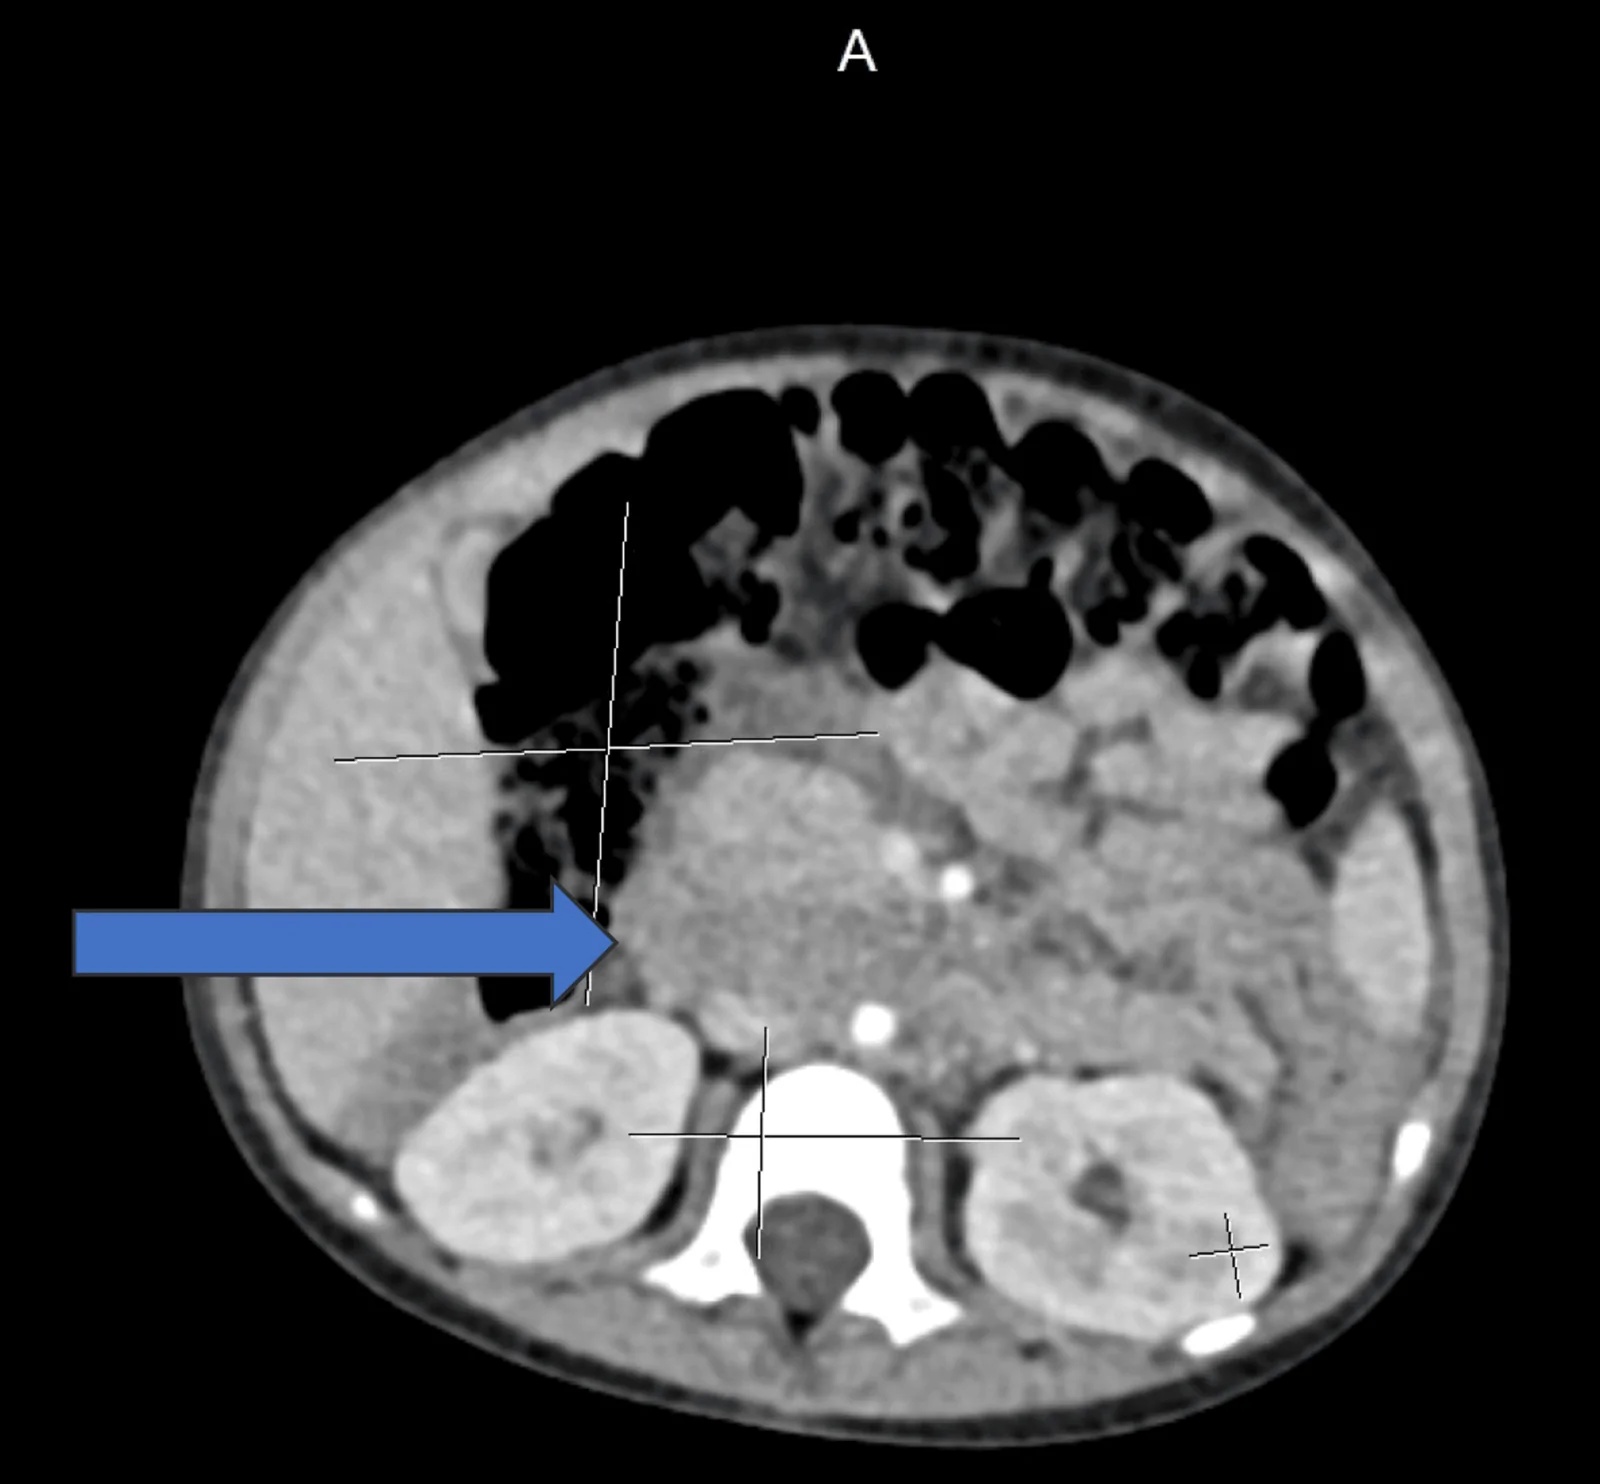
Contrast-enhanced CT scan demonstrating the primary intraabdominal mass during inpatient hospitalization CT: computed tomography
